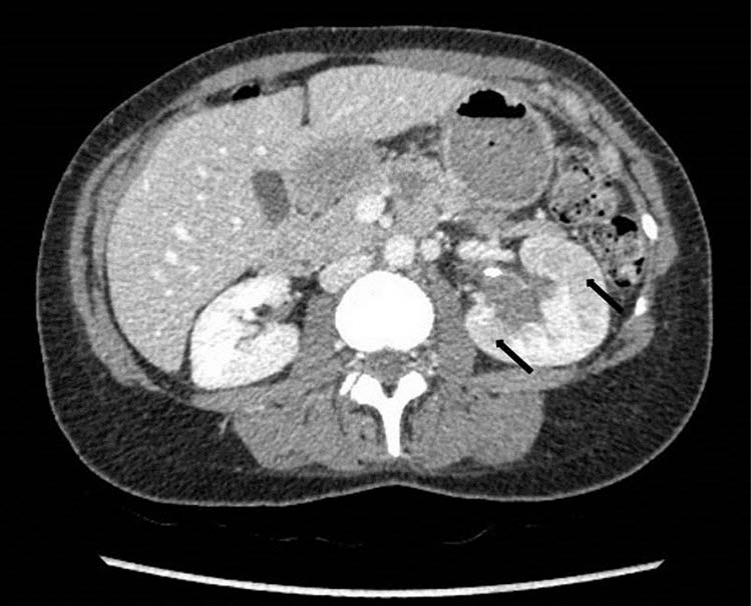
Computed tomography on presentation showing multifocal areas of hypoattenuation throughout the left renal cortex (arrows).
